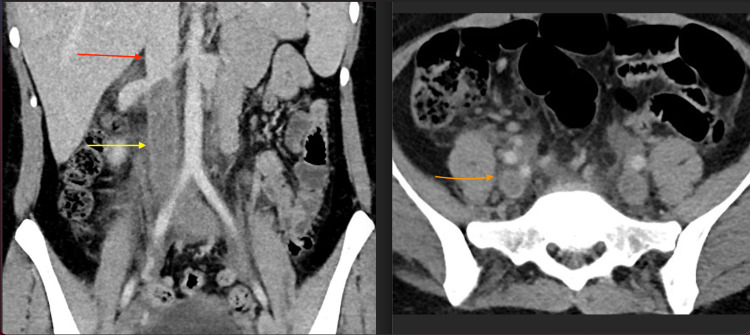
Coronal (left) and axial (right) contrast enhanced CT images show extensive thrombus within the infrarenal IVC (yellow arrow) extending into the right and left common iliac veins (orange arrow). The suprarenal IVC is patent (red arrow).
